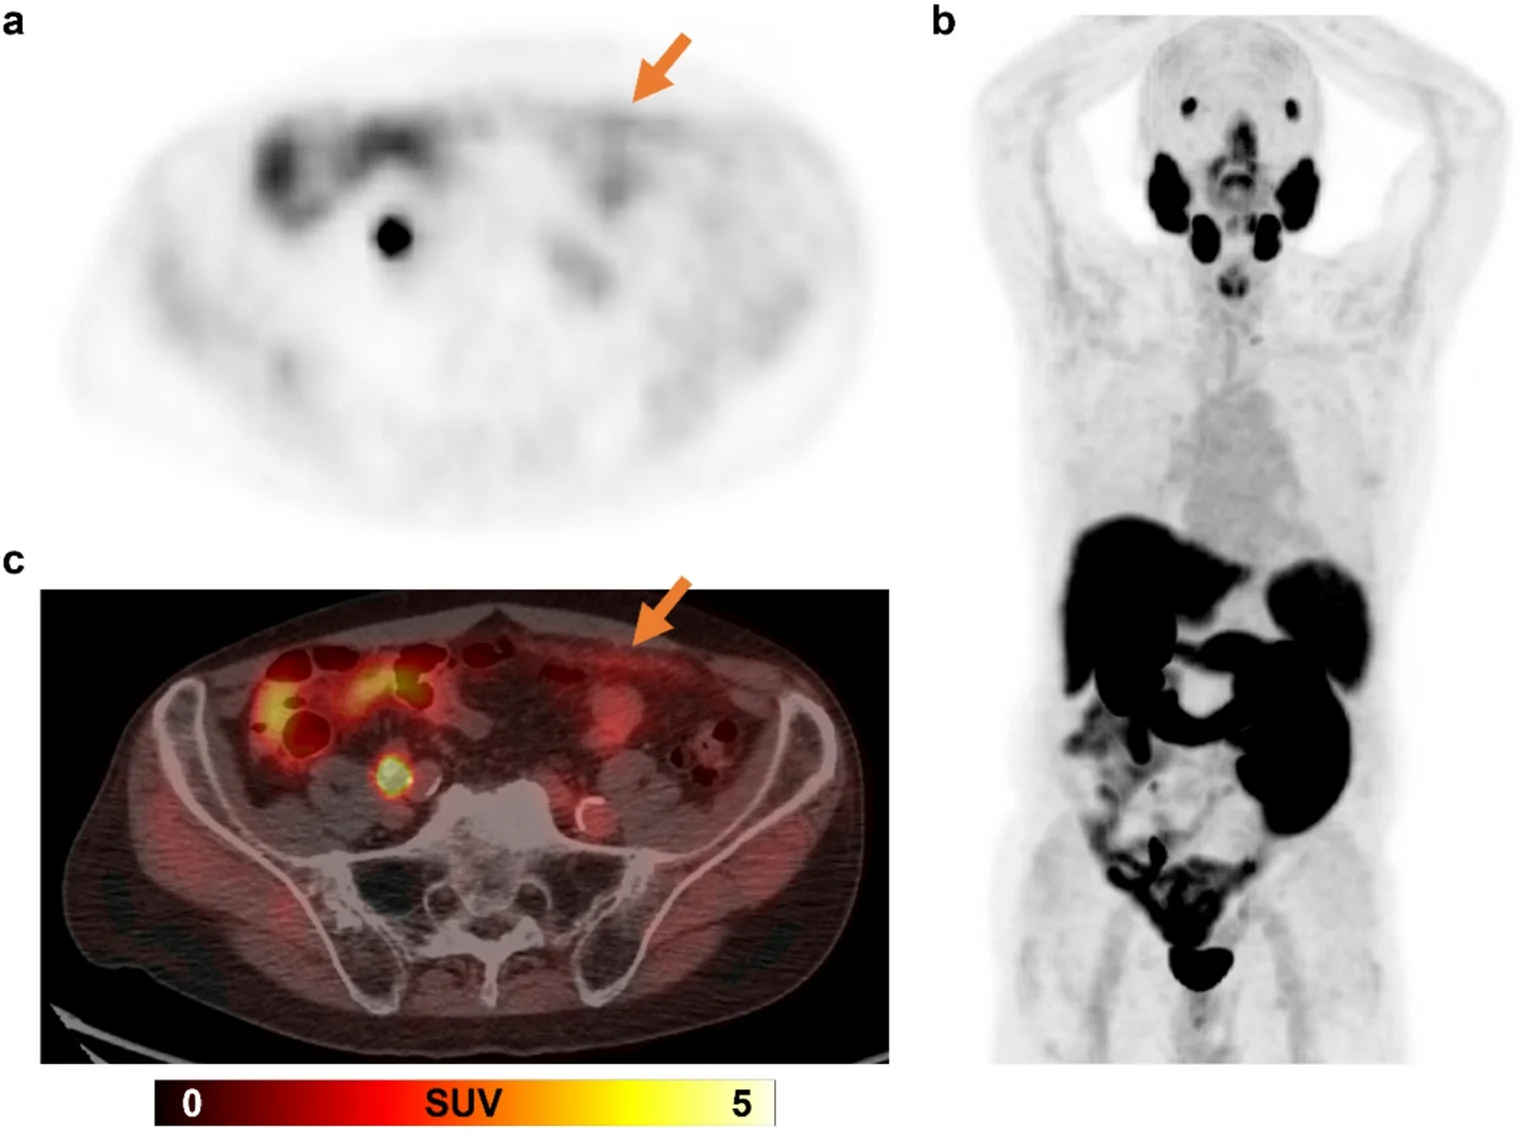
Patient with isolated PSMA-avid peritoneal metastases. 81-year-old man with prostate adenocarcinoma (Gleason 3 + 4) post-prostatectomy and intermittent ADT, with rising PSA (6.63 ng/mL). (a) Axial ^68^Ga-PSMA PET image shows linear avidity (SUVmax 2.5; arrow) in the left anterior lower quadrant, corresponding to omental nodularity (arrow) on axial fused PET/CT (b), without other sites of disease seen on maximum-intensity projection image (c)
